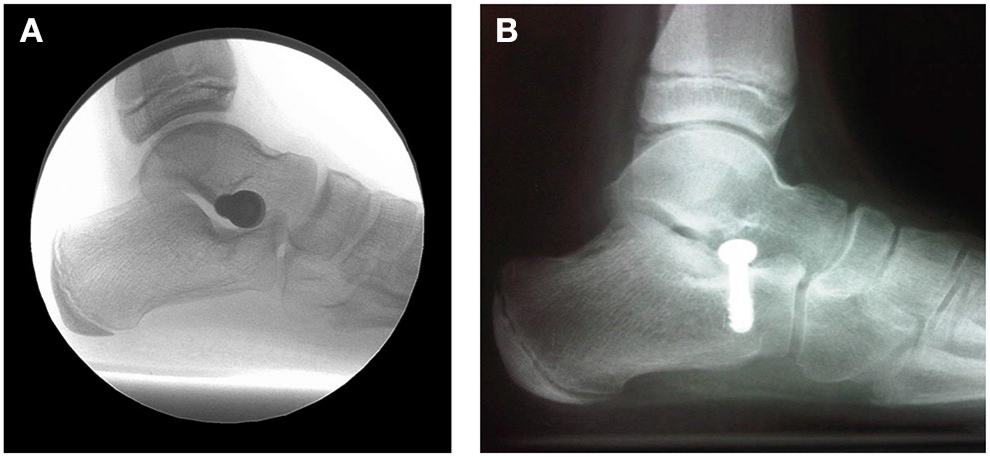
(A) Lateral weightbearing radiograph of an extra-osseous sinus tarsi implant. (B) Intra-osseous screw acting to stabilize the talotarsal joint.
